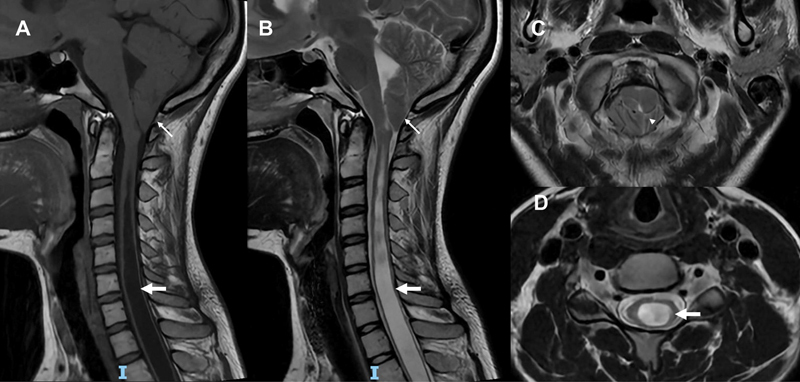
Magnetic resonance imaging scans of a patient with neuropathic upper limb pain showing Chiari I abnormality and syringomyelia. Sagittal T1-weighted (
